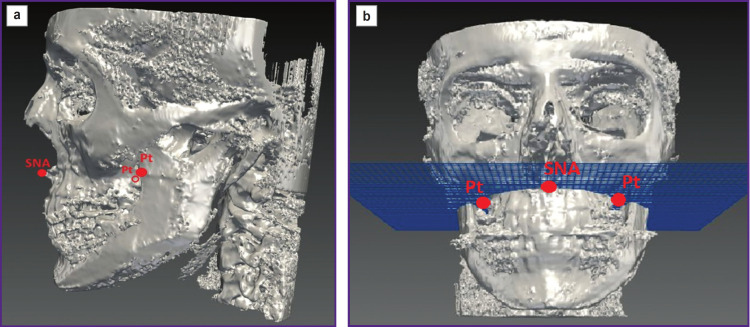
Determining the maxillary position according to Arkhipova and Arkhipov [76]: (a) the analysis of cone beam computed tomography image in the lateral view by constricting the plane going through the points SNA, Pt on the right and left; (b) the analysis of cone beam computed tomography image in the frontal view by constructing the plane going through the points SNA, Pt on the right and left
